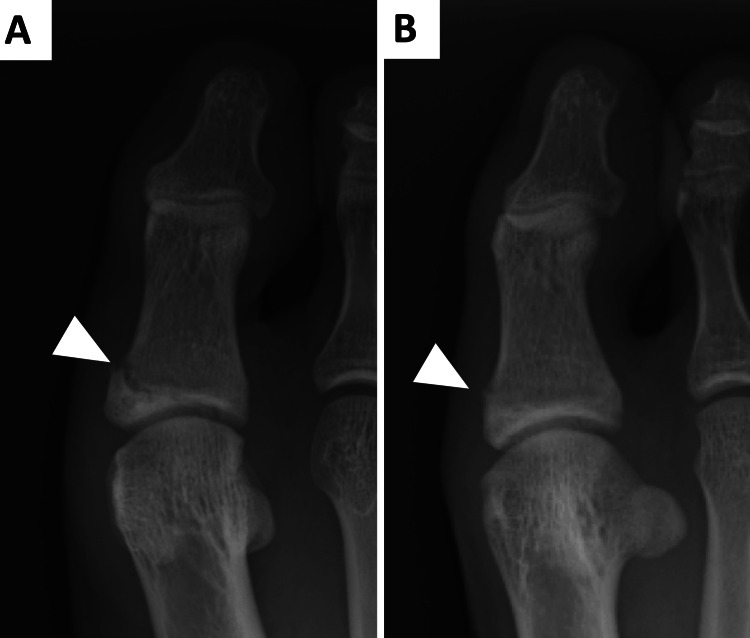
An X-ray at the time of the initial examination showed a crack at the medial base of the proximal phalanx of the great toe A: anteroposterior (AP) view; B: oblique view
